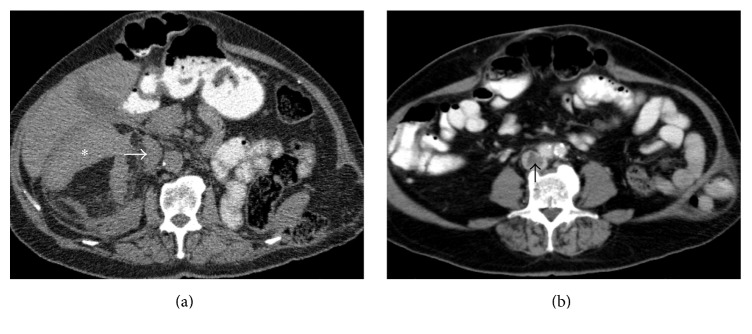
Axial CT images prior to intervention. (a) Unenhanced image shows the typical appearance of a large angiomyolipoma with fat and soft-tissue (*∗*) densities. The mass compresses the inferior vena cava (arrow). (b) Contrast-enhanced image (venous phase) shows a thrombus causing an enhancement defect (arrow) at the lowest part of the inferior vena cava.
